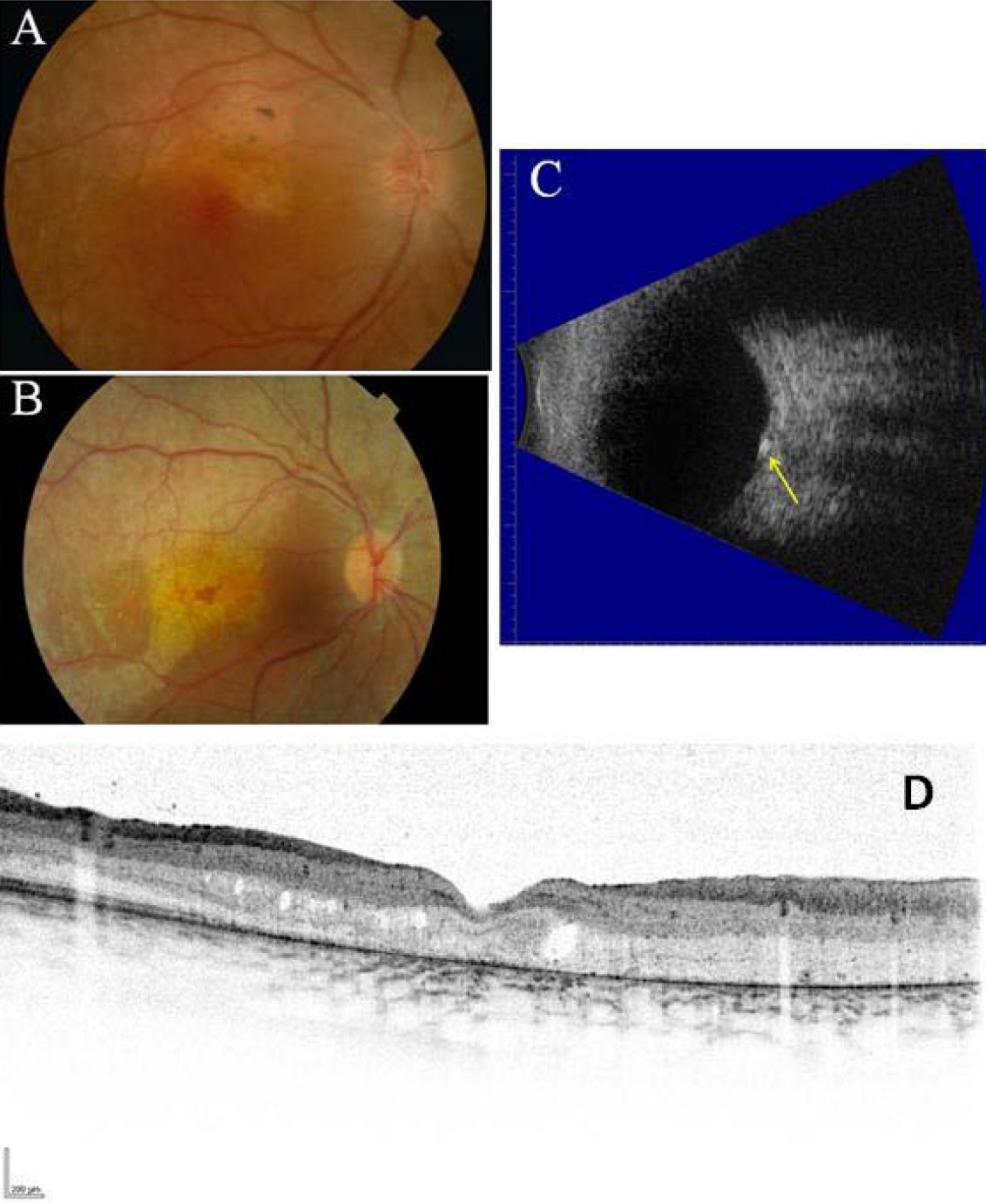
Ophthalmological images of two siblings affected by retinitis pigmentosa, nanophthalmus and optic disc drusen. Fundus photography of the right eye of patient IV:2 A: and of patient IV:3 B: showed atrophy of the retina outside the fovea and spots of hyperpigmentation. B-mode ultrasound of the left eye of patient IV:2 C: revealed optic disc drusen (indicated with the yellow arrow). Optical coherence tomography scan of the macula of patient IV:2 showed intraretinal edema and atrophy of the outer retinal layers D.
